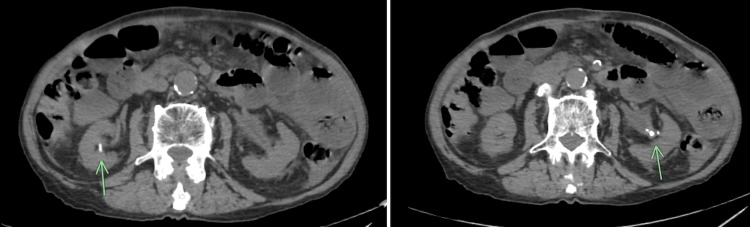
Abdominal and pelvic CT scan showing right and left nephrolithiasis CT: computed tomography
